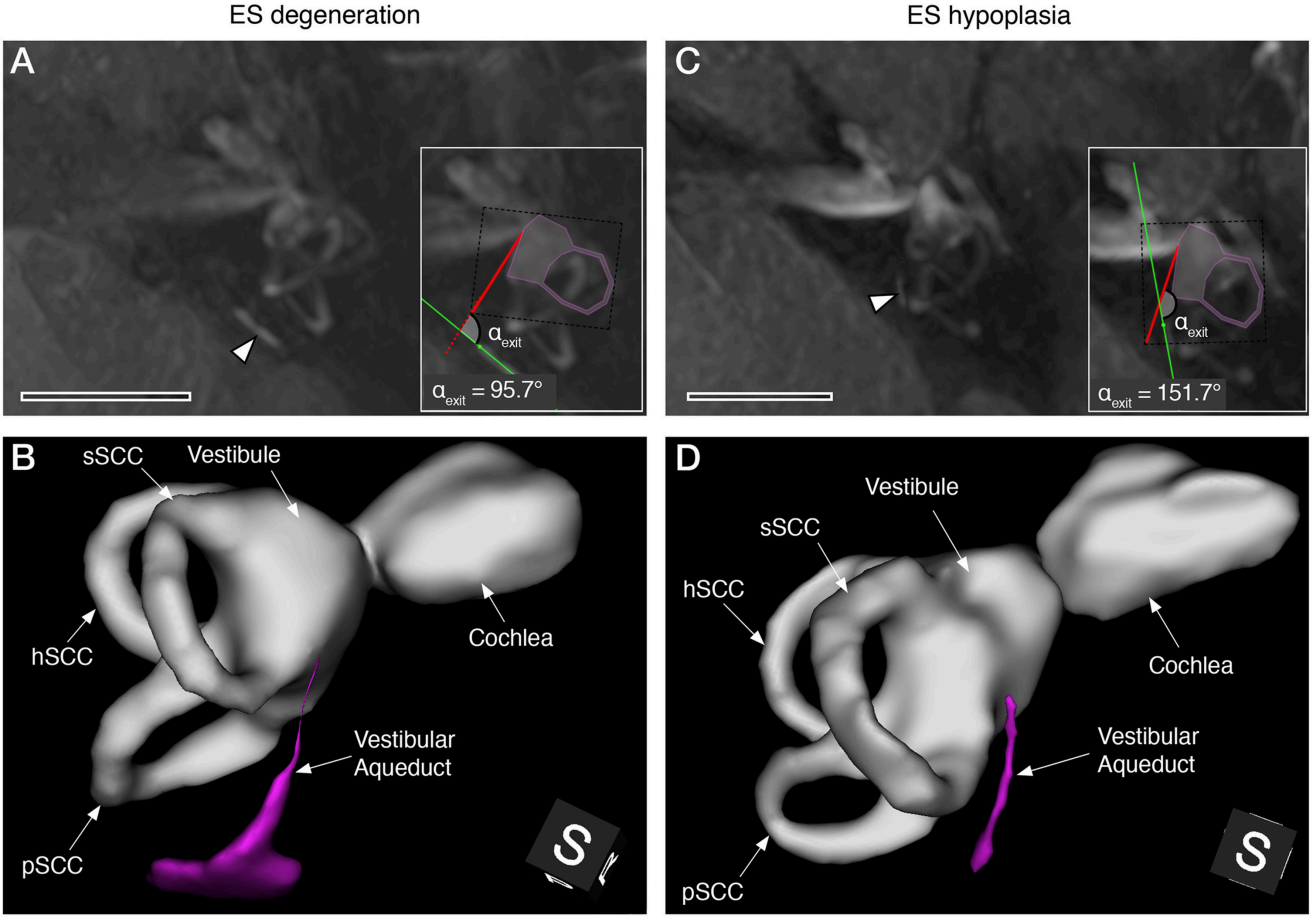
Gd-MRI VA morphology in representative MD cases with degenerative (A,B) and hypoplastic (C,D) ES pathology. Maximum intensity projections of axial plane images (A,C), and 3D reconstructions (postero-superior view) of the labyrinthine fluid spaces with the VA shown in magenta (B,D). Arrowheads in (A,C) indicate the VA in the opercular region. Insets in (A,C) show angle measurements of the VA's angular trajectory as described previously (7) (sSCC, superior semicircular canal; hSCC, lateral semicircular canal; pSCC, posterior semicircular canal). Scale bars, 10 mm.
